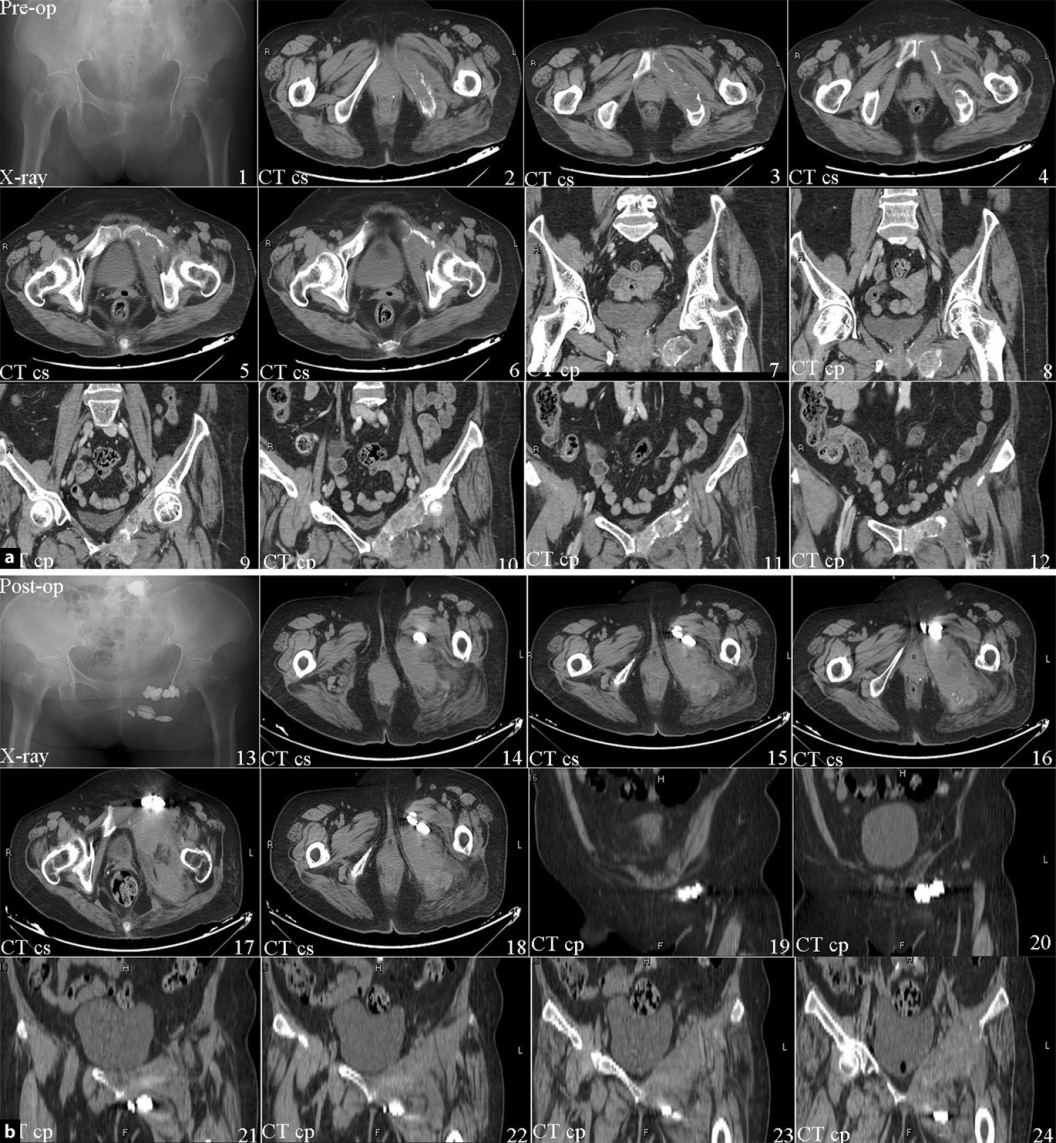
a *Pre-op* preoperative, b *post-op* postoperative. The preop CT shows the bone surface was sufficiently intact. The postop CT shows the bone cement filled the metastatic lesion and extruded into the soft tissues. *CT* *cs* computed tomography cross-section, *CT* *cp* computed tomography coronal plane
